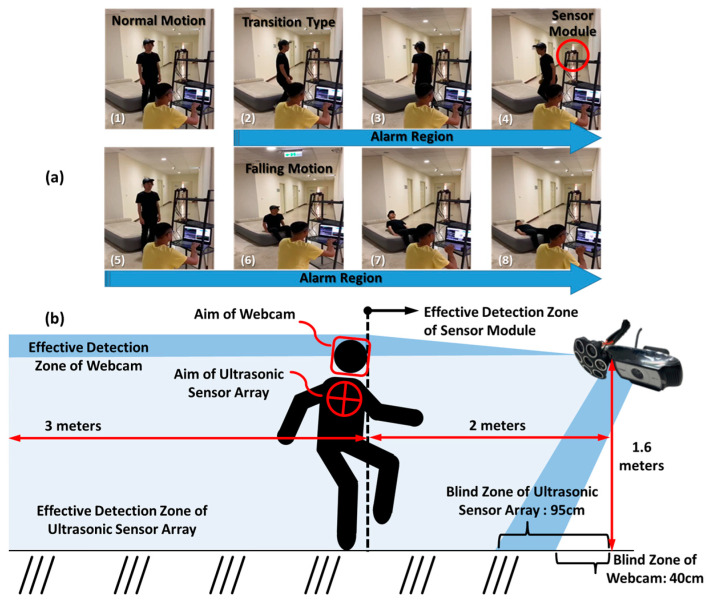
The series of images in (a) show the whole proposed experimental environment and falling stages. The sensing module was mounted on a shelf 1.6 m, and the data were collected and returned to the computer for model training. The alarm occurred when the hybridized platform sensed a transition. The image in (b) exhibits a detection-tracking scene of the proposed sensing scheme. Critical dimensions of effective and blind zones are clearly delineated. The targets of the ultrasonic sensor array and webcam were human chests and faces, respectively.
